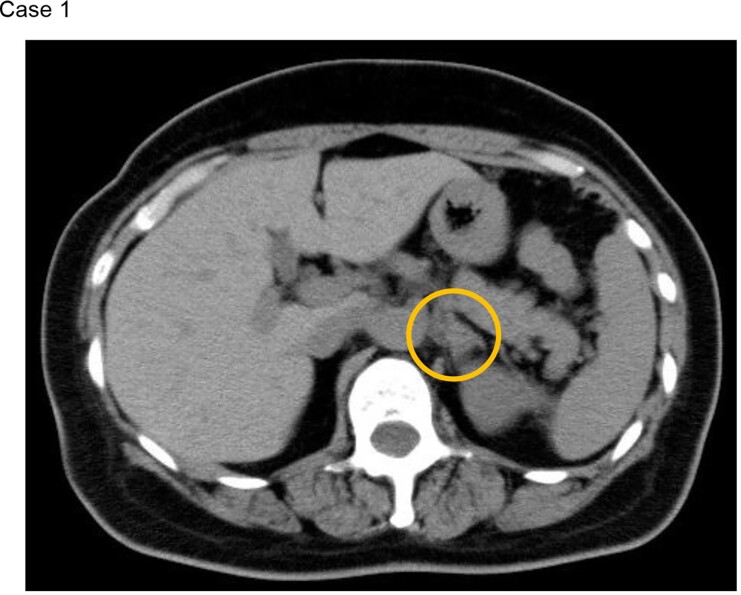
Image findings at the time of recurrence (case 1). The image shows computed tomography at age 52. The orange circle indicates a tumor with a diameter of 20 mm in the left adrenal gland.
